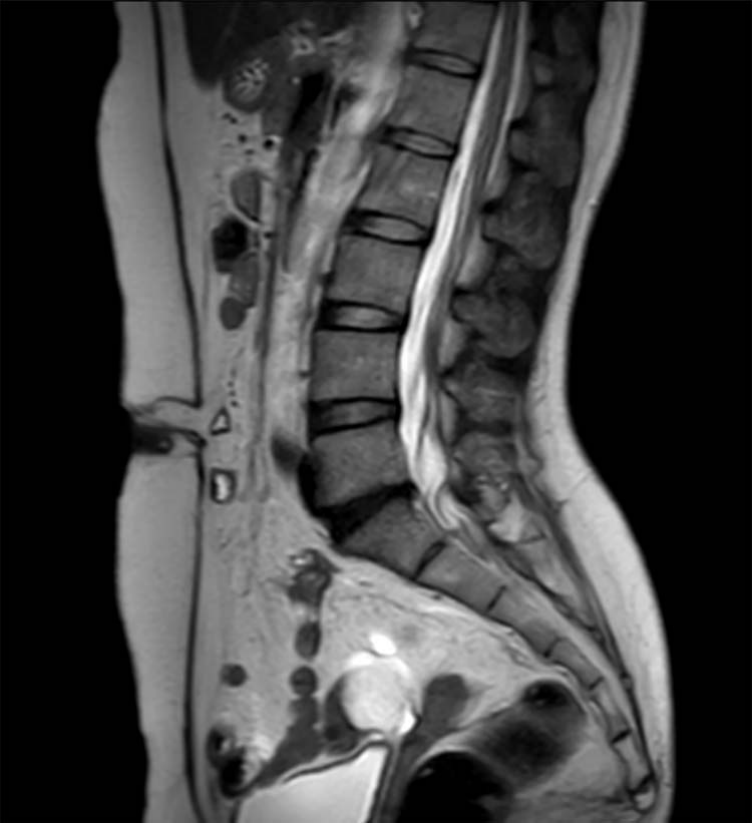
MRI sagittal view of the port site hernia.
